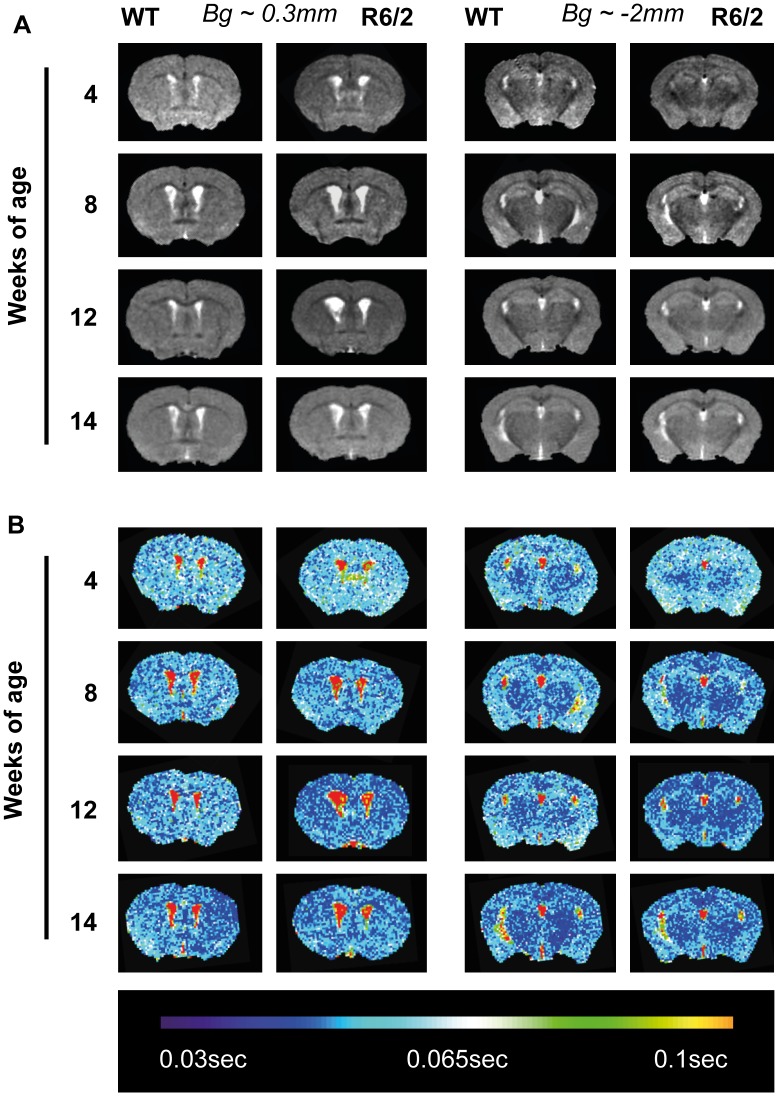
In vivo* MRI sample images. (A) Sample T2-weighted images for structural volumetry assessments. Images are from scans taken at 4, 8, 12 and 14 weeks of age at anatomically matching slices. (B) Hot/cold scaled maps of T2 relaxation times for the study of T2 relaxivity, these are represented on anatomically identical images to those in (A). Bg = bregma.
